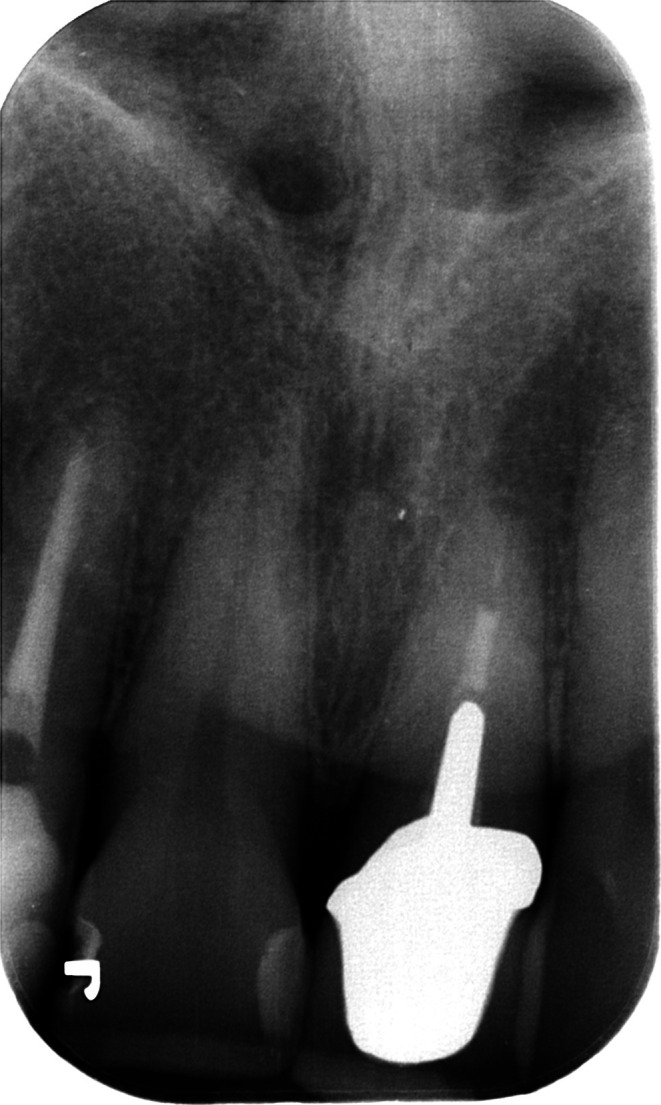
Initial radiographic examination.
